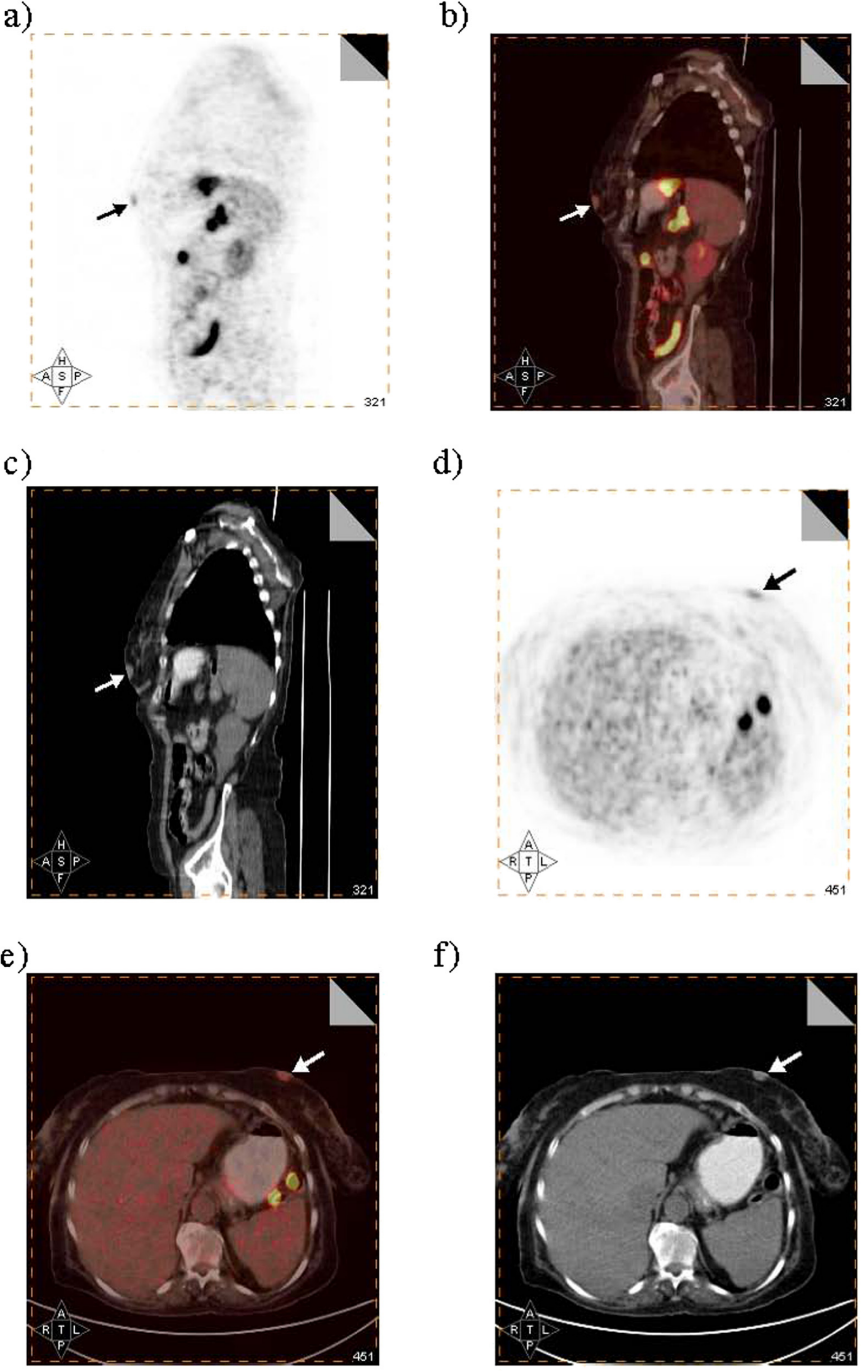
Sagittal (a, b, c) and axial (d, e, f) positron emission tomography-computed tomography images demonstrating a small focal region of moderate fluorodeoxyglucose uptake within the left breast (arrows) compared to normal parenchyma.
